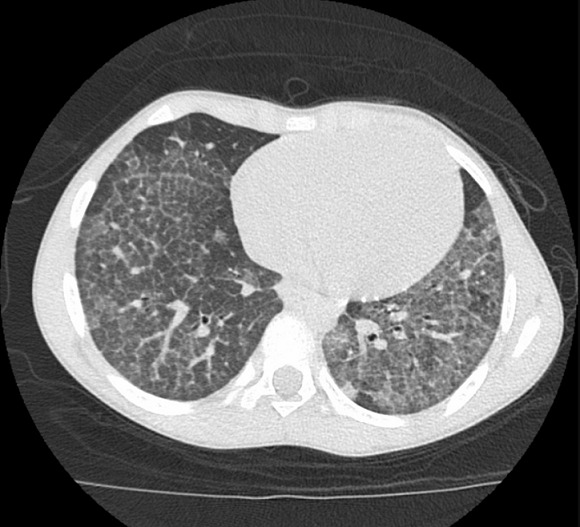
HR-CT scan showing diffuse ground glass opacities indicative of alveolar haemorrhage and thickened interlobar septae
